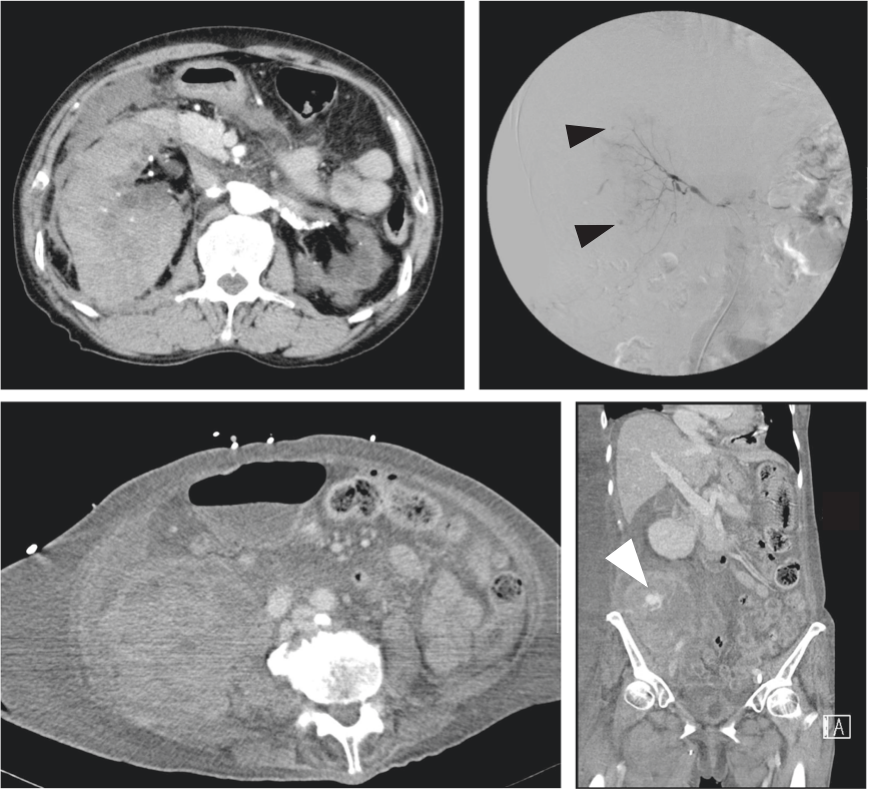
Computed tomography and angiographic images in cases 3 and 4. Upper left panel shows an abdominal CT image from case 3. A giant intramuscular hematoma in the right iliopsoas muscle is shown. Upper right panel shows an angiographic image from case 3. Extravasation was found in the 4th lumbar artery (black arrowheads). Lower panels show abdominal CT images from case 4 (left: axial view, right: coronal view). A giant retroperitoneal hematoma and the active bleeding point (white arrowhead) are shown.
